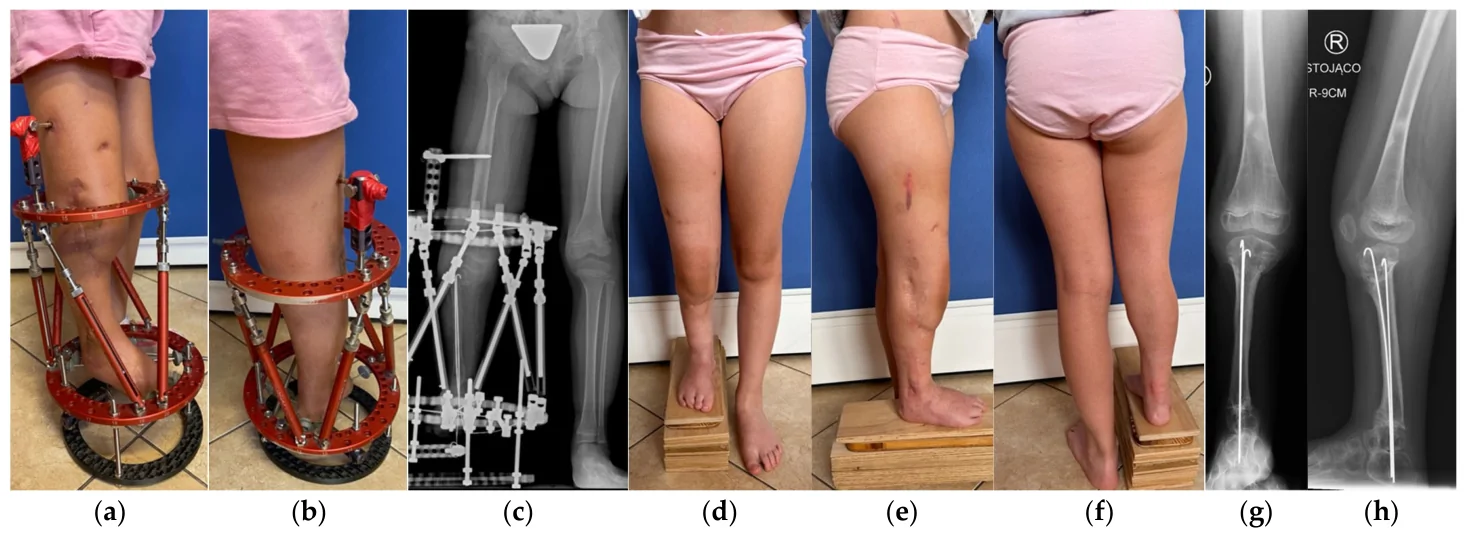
Foot correction and stabilization using an external frame: (a,b) clinical and (c) radiographic view; (d–f) clinical and (g,h) radiographic result. PKD—right lower limb, NA STOJĄCO—standing position, R—right.
